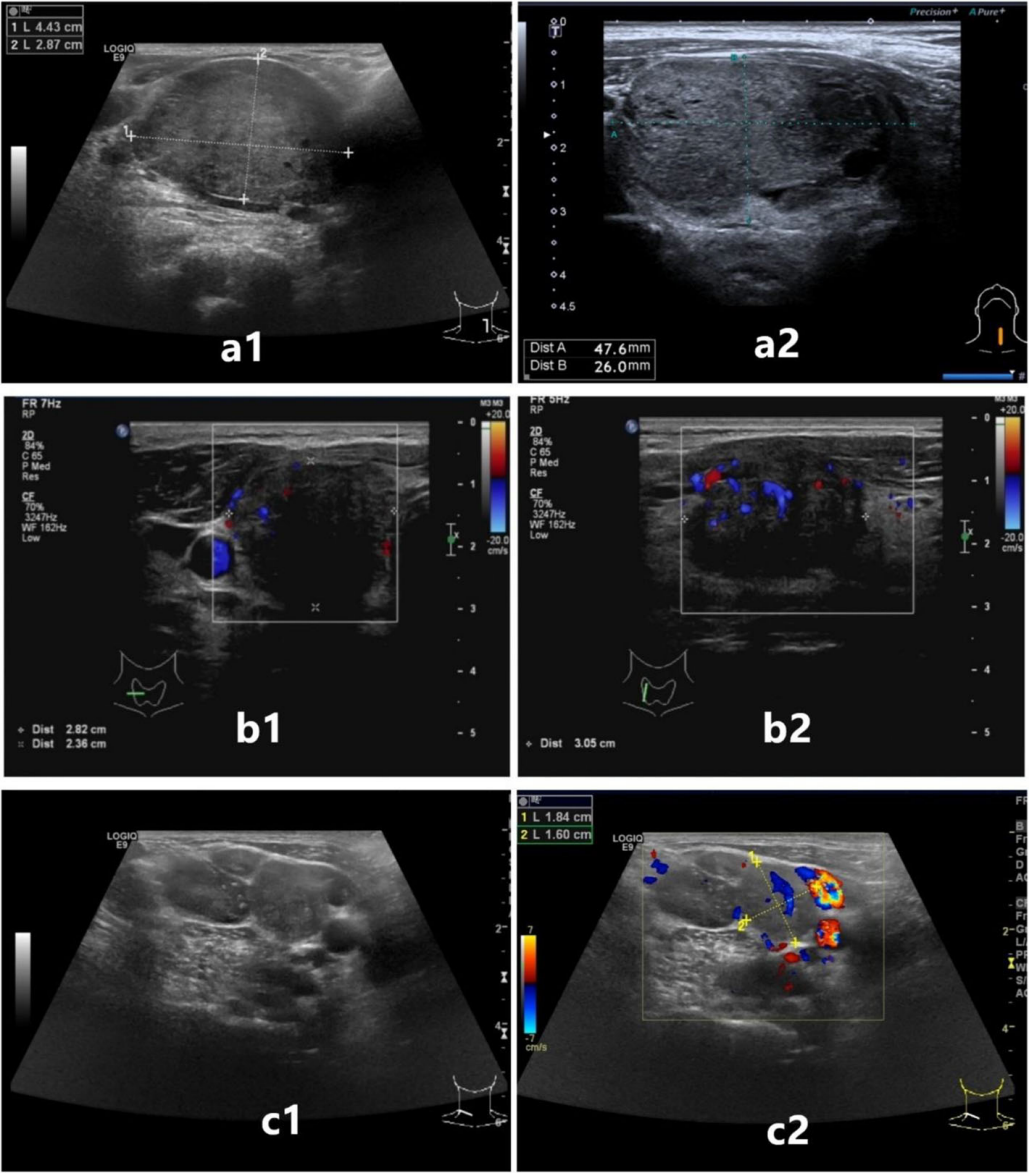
Ultrasonic characteristics of the MTC tumor: a1 and a2 show tumor size> 40.0 mm; b1 and b2 show capsular invasion; c1 and c2 show metastatic cervical lymph nodes: the lymph nodes appear enlarged, round
